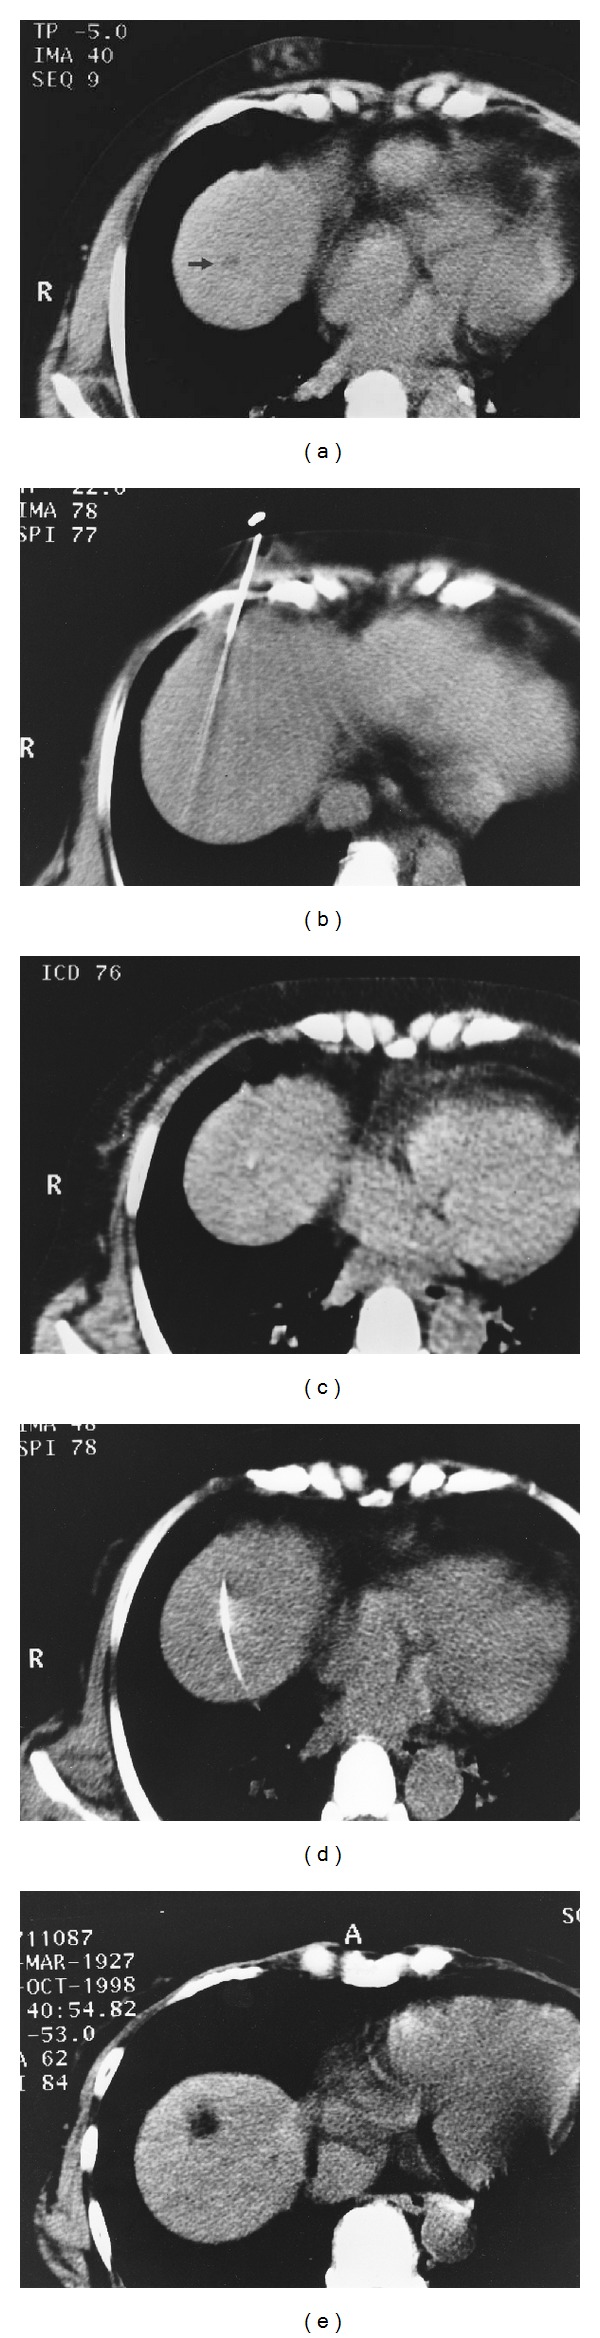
Computed-tomography- (CT-) guided needle approach using a directable needle guide (DNG) for percutaneous ethanol injection. (a) CT image (with tilt of the gantry 33° in the cranial direction) of a 73-year-old woman with hepatitis C shows a hypodense, growing lesion (arrowhead) in the hepatic dome. (b) A 22-gauge injection needle is initially inserted at a position caudal to the CT image plane, avoiding insertion into lung tissue. (c) A DNG (arrow) is advanced in the craniomedial direction to penetrate the lesion under intermittent fluoroscopy. (d) The injection needle is advanced along the DNG. (e) CT image (after replacing the gantry) demonstrates a lesion with decreased attenuation after ethanol injection.
